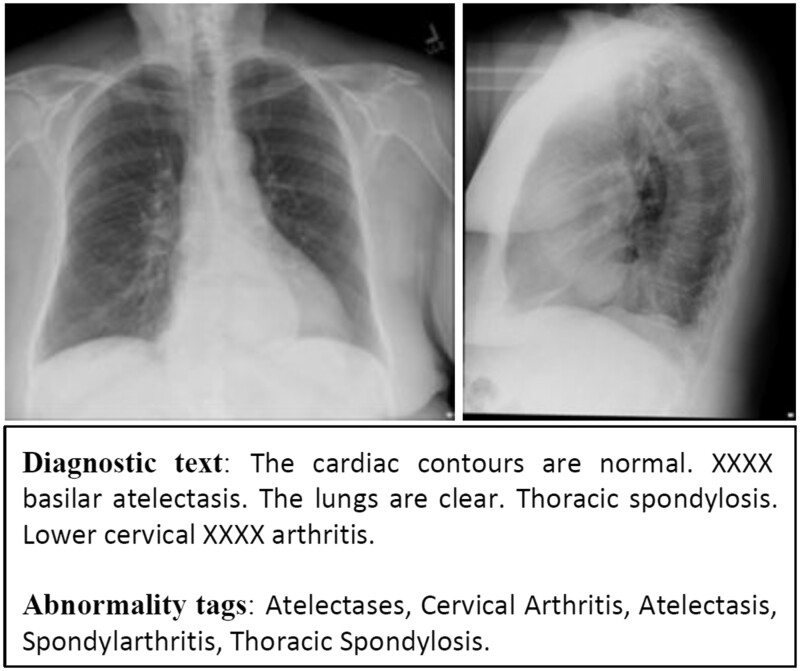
A posteroanterior/lateral chest radiography exam along with the corresponding human-authored DIAGNOSTIC TEXT from IU X-ray, and the abnormality tags. The “XXXX” is due to the de-identification process.
